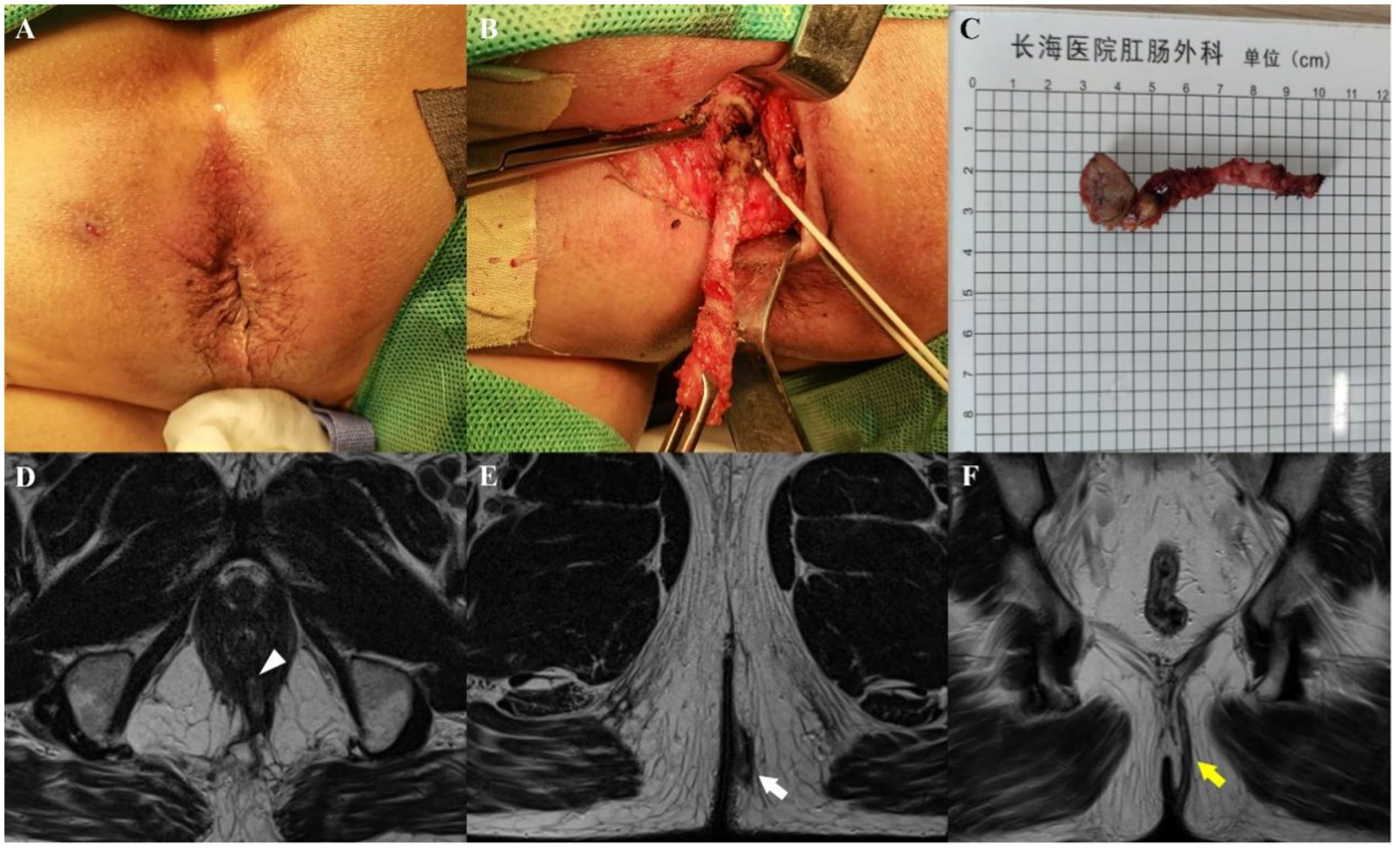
A 62-year-old man whose perianal purulent secretion had persisted for 6 months. Preoperative appearance (A) and findings during the operation (B) showing a suprasphincteric fistula. (C) Sample from surgical resection. Axial T2-weighted images without fat suppression show a left transsphincteric fistula crossing both layers of the sphincter complex, with an internal opening at the 6 o’clock position (D, arrowhead) and an external opening at the 5 o’clock position (E, arrow). Coronal T2-weighted image without fat suppression (F) showing upward fistula extensions through the intersphincteric space, over the top of the levator ani muscle, and then descending through the ischiorectal fossa at the left side to reach the skin (yellow arrow). Preoperative MRI findings demonstrated complete concordance with intraoperative exploration.
